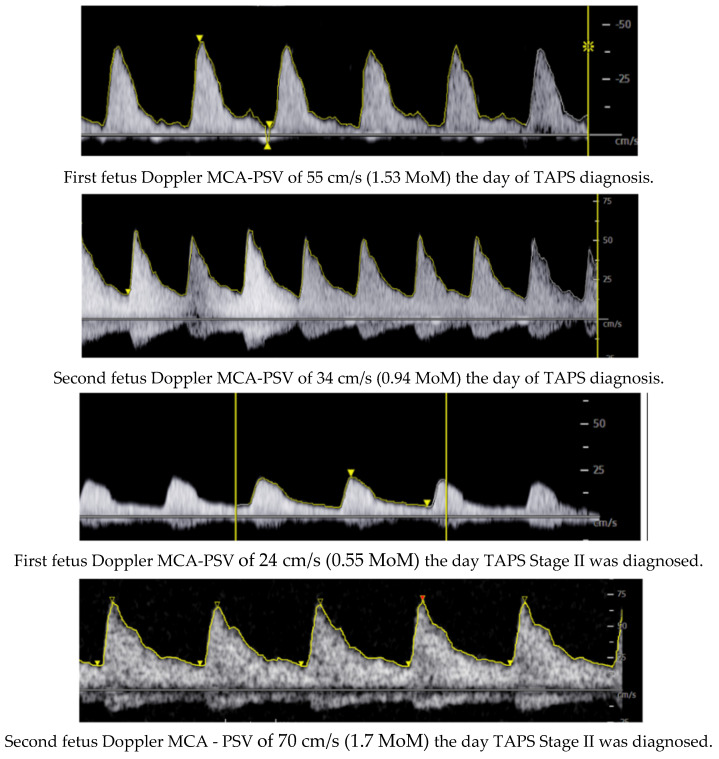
Doppler ultrasonography of evolution of MCA-PSV of the twins.
